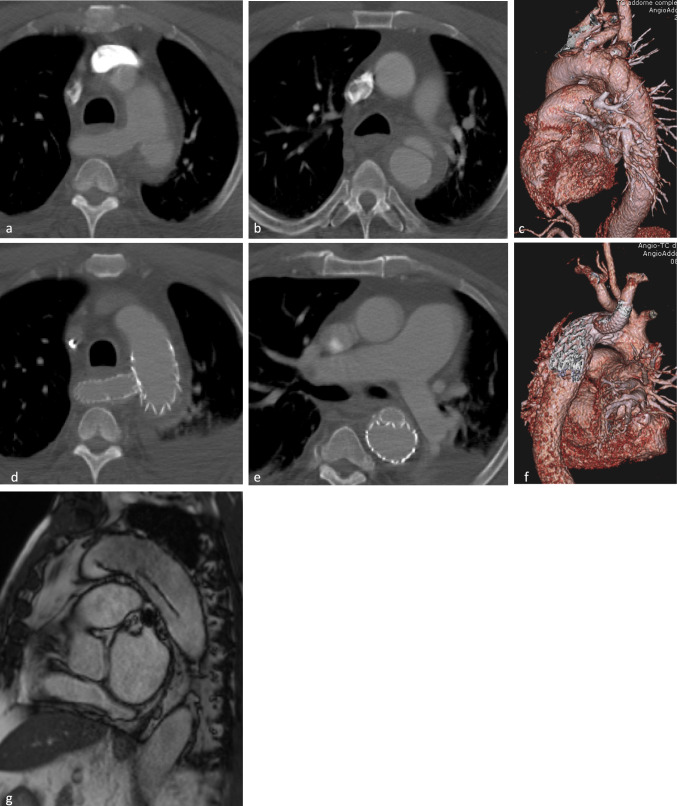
Grade III aortic isthmic lesion in ARSA anatomical variant treated with Gore C-TAG 36 × 150 mm and stent (Viabahn 13 × 100 mm) in ARSA, placed according to periscope technique. a b Preoperative CTA and c volume rendering (VR) reconstruction; d e postoperative CTA and f VR: correct placement and patency of aortic endoprotestheses; g 108-months follow-up MRI: no prostheses- and stent- related complications
